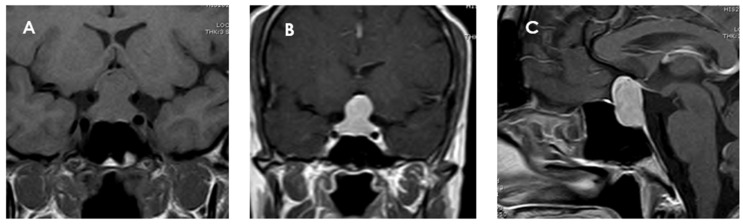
Magnetic resonance imaging of a pituicytoma (PTC). The lesion measures 25 × 12 × 14 mm (cranial–caudal × anteroposterior × transverse), with superior displacement of the optic chiasm and discrete compression of the pituitary stalk. (A) Coronal T1 sequence without contrast showing an isointense mass located in the sellar and suprasellar region. (B) Coronal T1-weighted coronal section with gadolinium showing homogeneous enhancement of the lesion. (C) Sagittal T1-weighted contrast-enhanced sagittal section confirming the suprasellar location of the mass, well delimited and with intense contrast uptake, and with no signs of invasion to adjacent structures.
